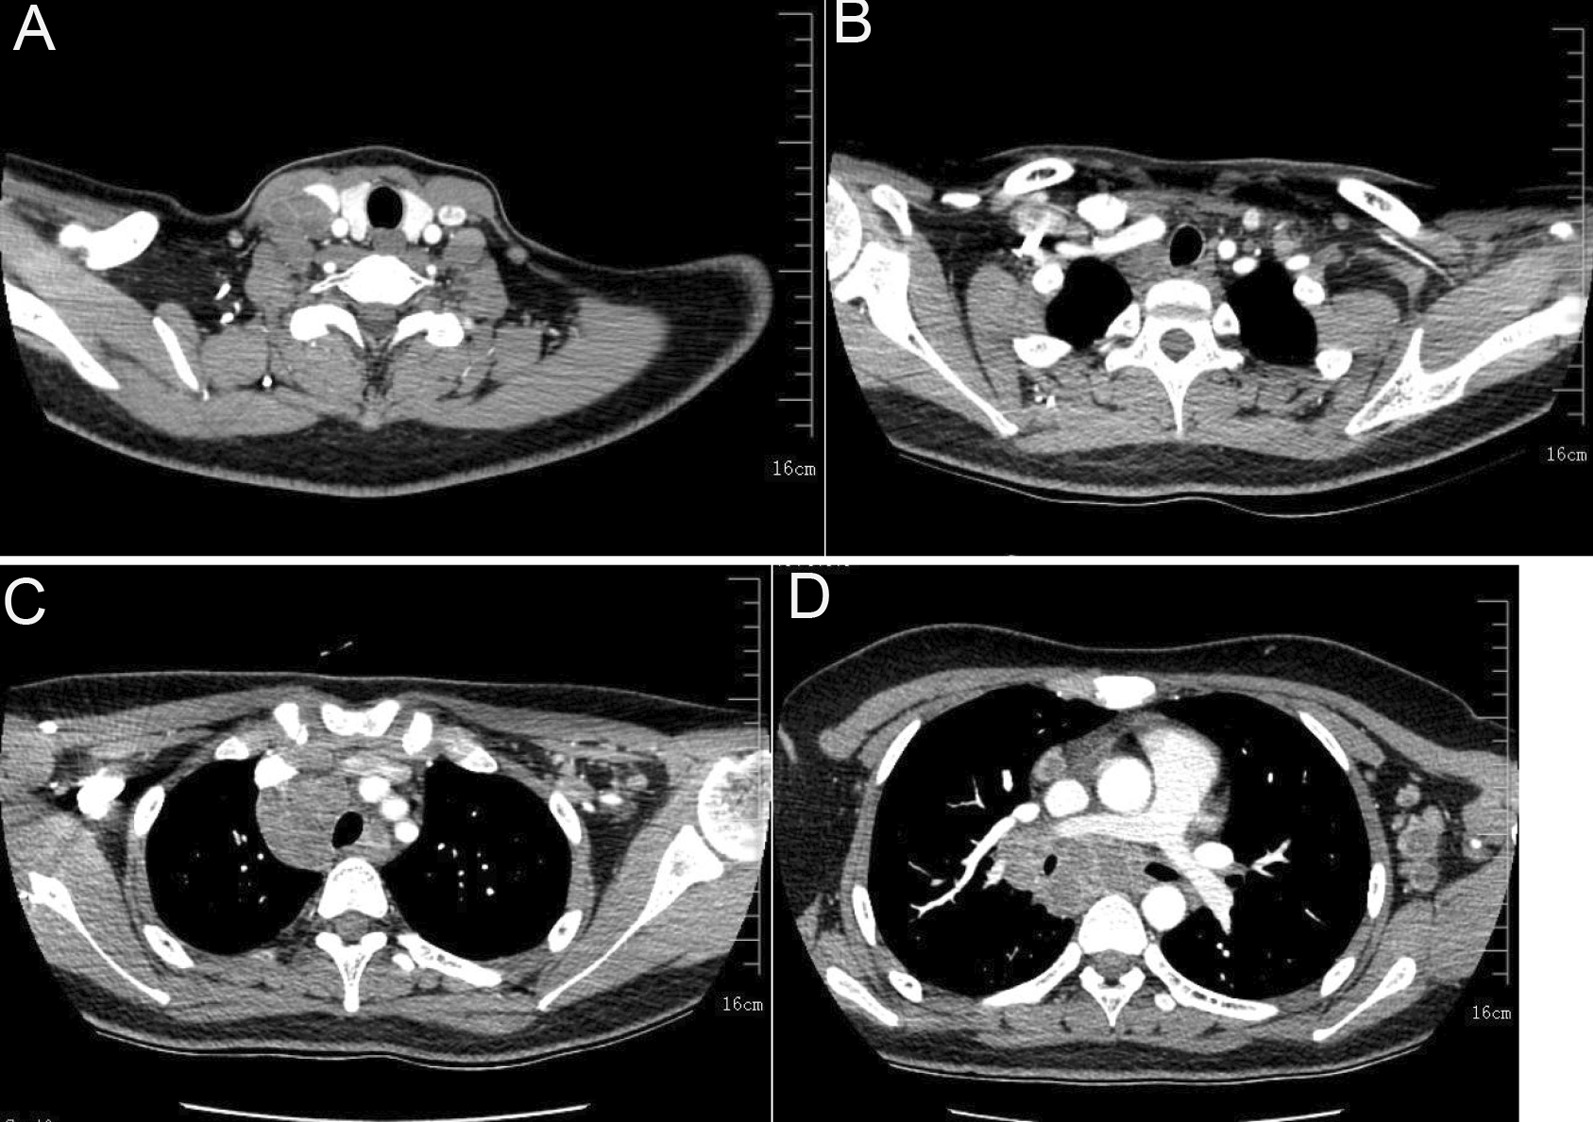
Contrast-enhanced CT of the neck. A Liquefaction in the adhesion and fusion regions of cervical lymph nodes. B Right supraclavicular lymph nodes with necrosis in their center. C Necrosis in the adhesion and fusion regions of anterior mediastinal lymph nodes. D Uneven density in the adhesion and fusion region of posterior mediastinal lymph nodes
